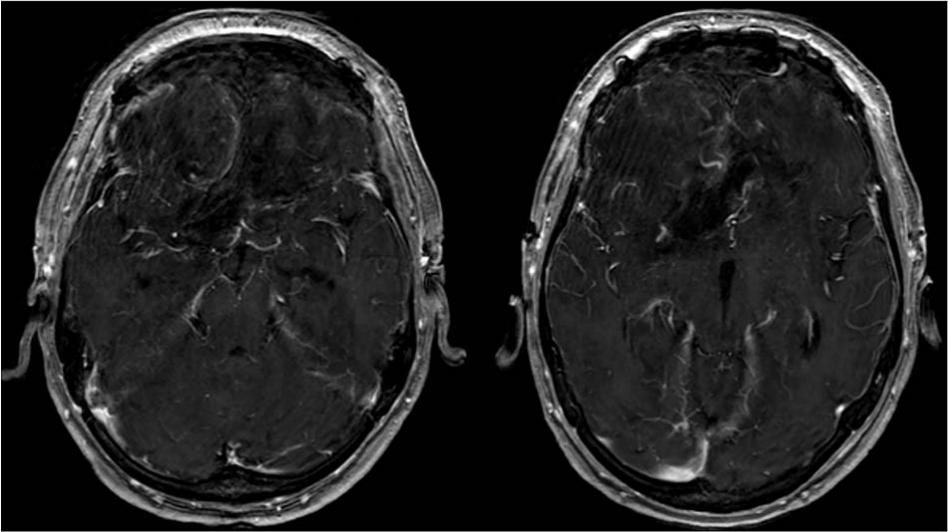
Showing the postoperative T1-weighted MRI with no residual tumor tissue.
